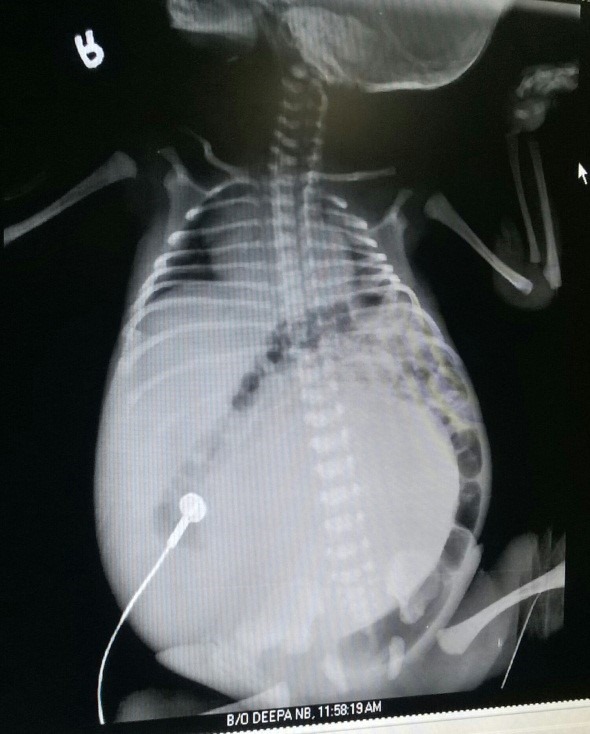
Figure 1: X-ray abdomen showed a huge homogenous soft tissue opacity occupying almost whole abdomen pushing gut to the left side.
